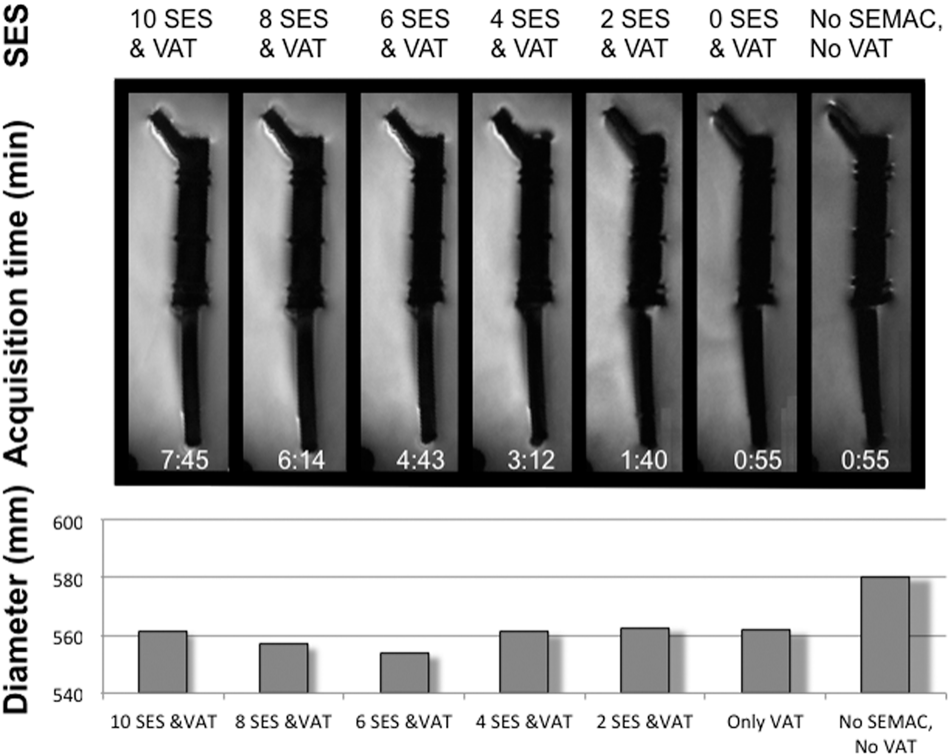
Reduction of slice-encoding steps (SES) for slice-encoding metal artifact correction (SEMAC). SES were reduced from 10 to 0 and assessed quantitatively and qualitatively. No SEMAC is equivalent to 0 SES. Acquisition time for all SES values is shown in the images. For qualitative evaluation, artifact diameters (mm) were measured on all MR images. VAT, view-angle tilting. SEMAC; slice-encoding metal artifact correction.
